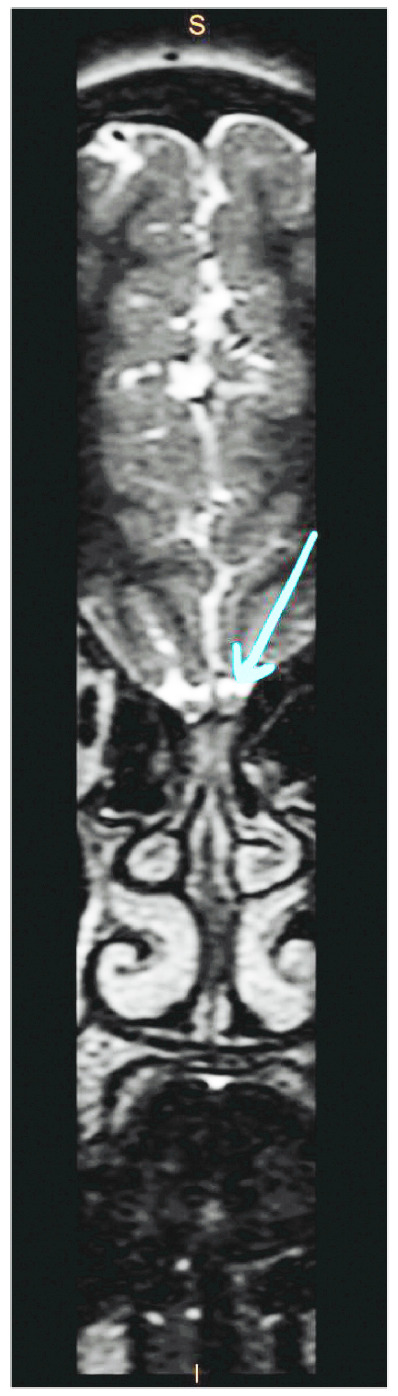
Coronal view of the MRI brain with olfactory protocol showing normal volume of olfactory bulbs (blue arrow).
